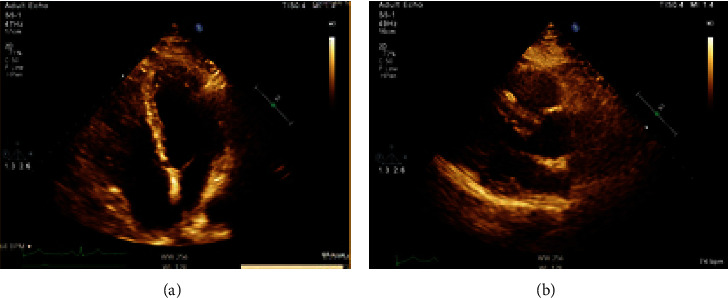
(a) Selected image of transthoracic echocardiogram in apical 4 chamber view and (b) a parasternal long axis view showing marked interval improvement of the pericardial thickening with resolution of the pericardial effusion.
